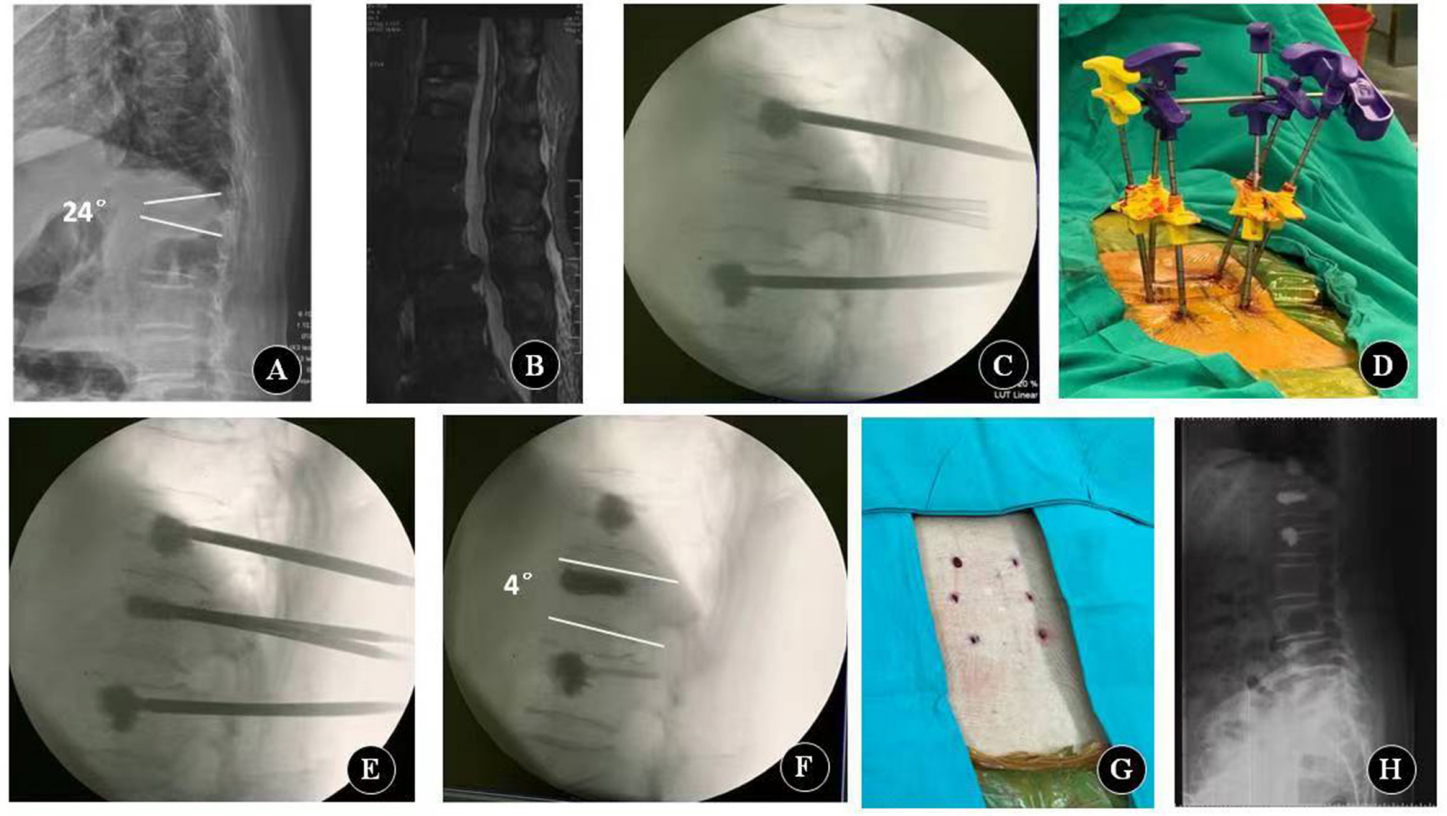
Key procedures of percutaneous lordcoplasty. (A) Preoperative lateral X-ray. (B) Preoperative sagittal MRI. (C) Reinforce adjacent vertebral bodies with cement. (D) Relevant reduction of the fractured vertebra is performed by a lordosing force. The reduction force of the cannulas is held with a cross blunt trocar. (E) Fractured vertebra body is augmented with cement. (F–H) Fluoroscopy image and appearance after the cannulas are removed.
